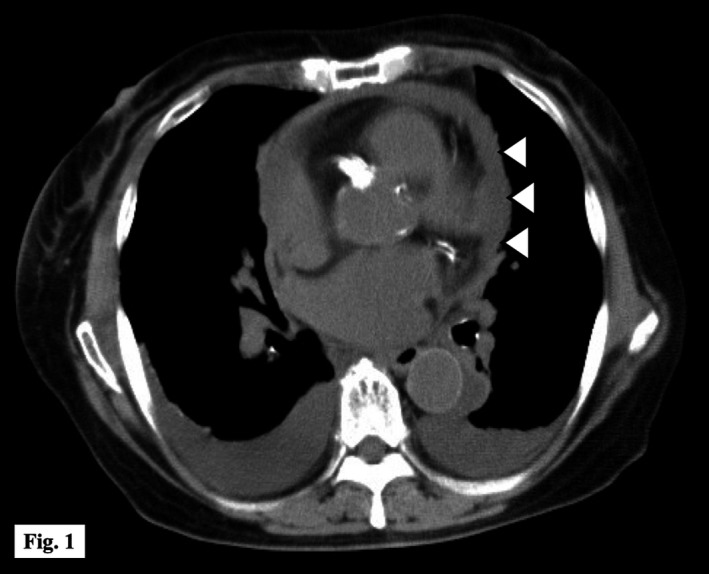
Noncontrast chest CT on admission demonstrated a small volume of pericardial effusion (white arrowhead)
